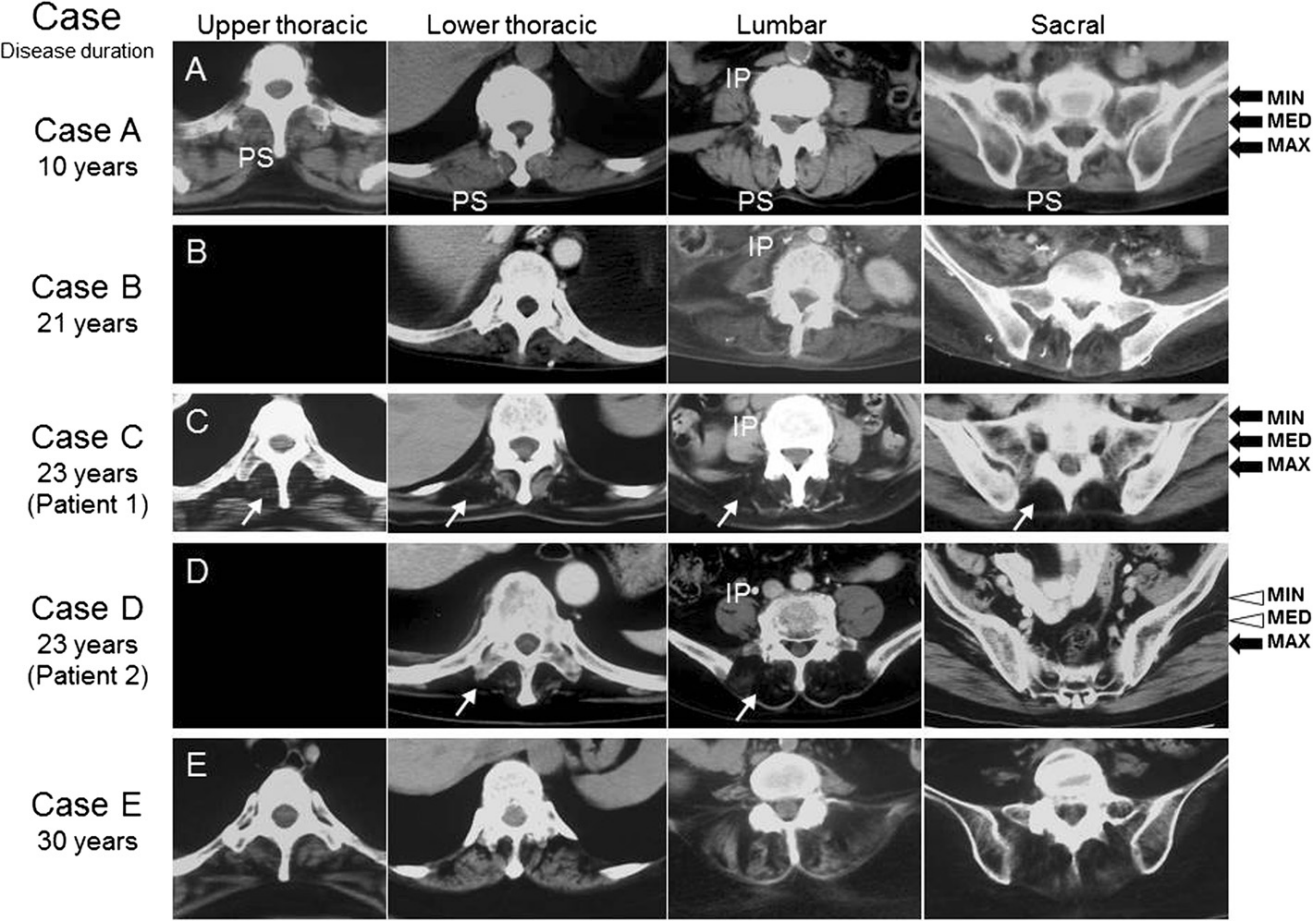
Atrophy in paraspinal muscles of the siblings. (A-E) Computed tomography studies of 5 patients with HAM/TSP at several truncal levels (upper thoracic, lower thoracic, lumbar and sacral levels). (A, B, E) The degree of muscle atrophy of the control HAM/TSP patients was in accordance with the duration of HAM/TSP. (C, D) The two siblings showed extreme atrophy of the paraspinal muscles (arrow) in comparison to the iliopsoas muscles (IP). The difference in atrophy between iliopsoas and paraspinal muscle of the two siblings is conspicuous in comparison to case B with similar disease duration. (D) The gluteus medius and minimus (arrow head) were also selectively atrophied in the elder brother. PS, paraspinal muscles; IP, iliopsoas; MAX, gluteus maximus; MED, gluteus medius; MIN, gluteus minimus.
